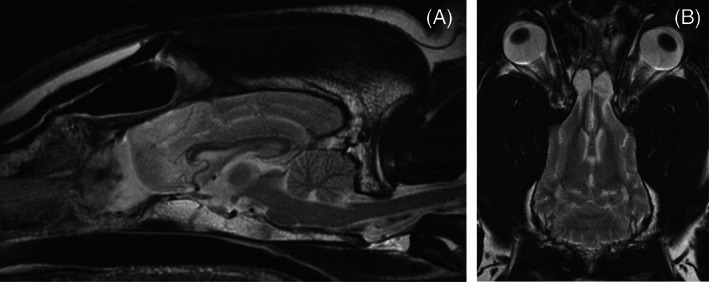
Six month postoperative sagittal (A) and dorsal (B) T2‐weighted MRI images.
